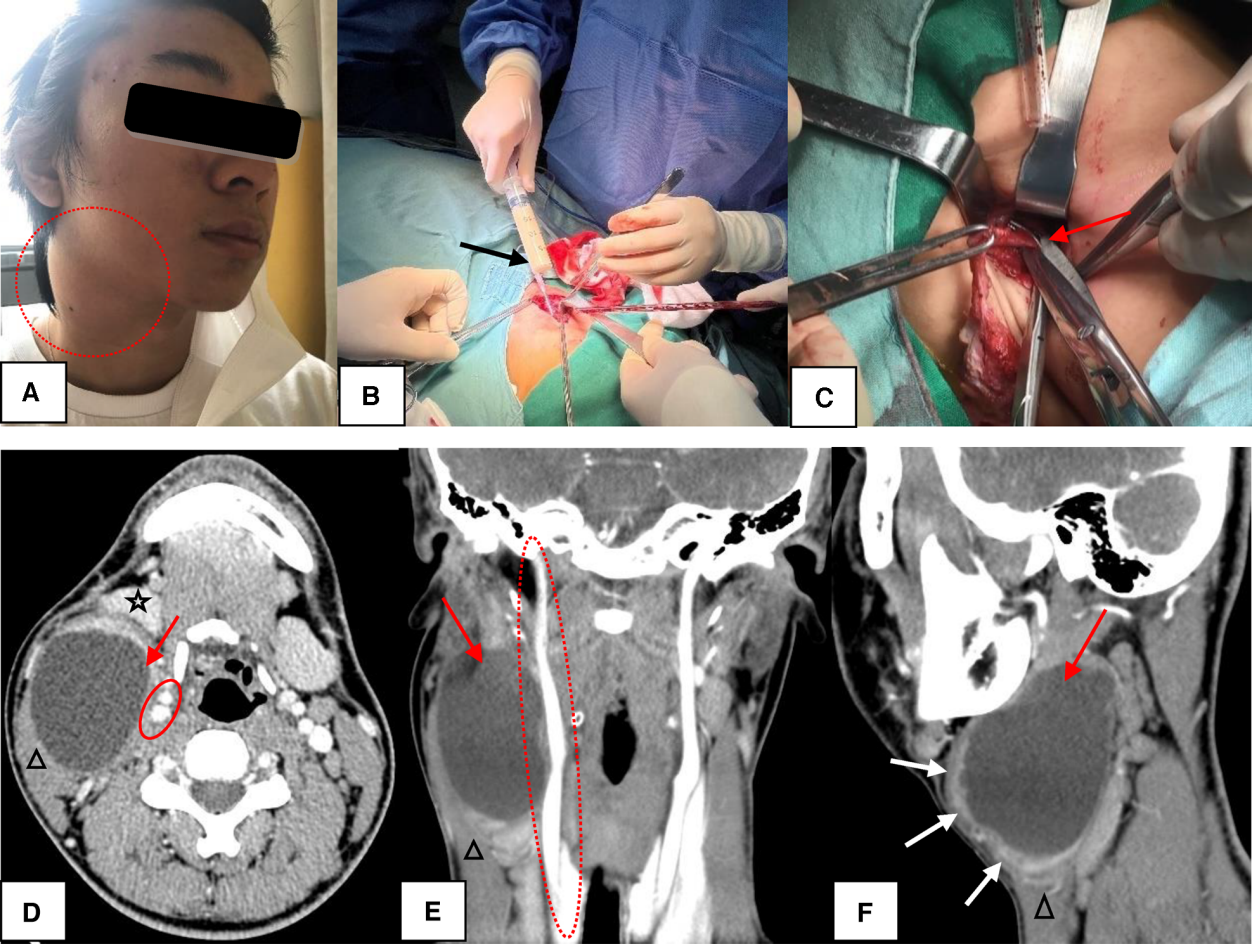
The Bailey type II. (A) The lesion presented as a painful mass in the right neck (circle). (B) During the operation, the mass contained a large amount of rice soup like liquid (black arrow). (C) High ligation of the sinus (red arrow) was sufficient for complete excision of CSBCAs. (D–F) CT scans showed a well-circumscribed and homogeneously low-density cystic mass (red arrow) with uniformly thin, smooth wall. If infected, the cyst wall became thickened and irregular (F, white arrow). The lesion was found in the “classic” location: along the anteromedial border of the SCM (triangle), lateral to the carotid sheath (circle), and posterior to the SMG (five-pointed star). D, axial position, E, coronal position, F, sagittal position; CSBCAs, congenital second branchial cleft anomalies; SCM, sternocleidomastoid muscle; CT, computed tomography.
